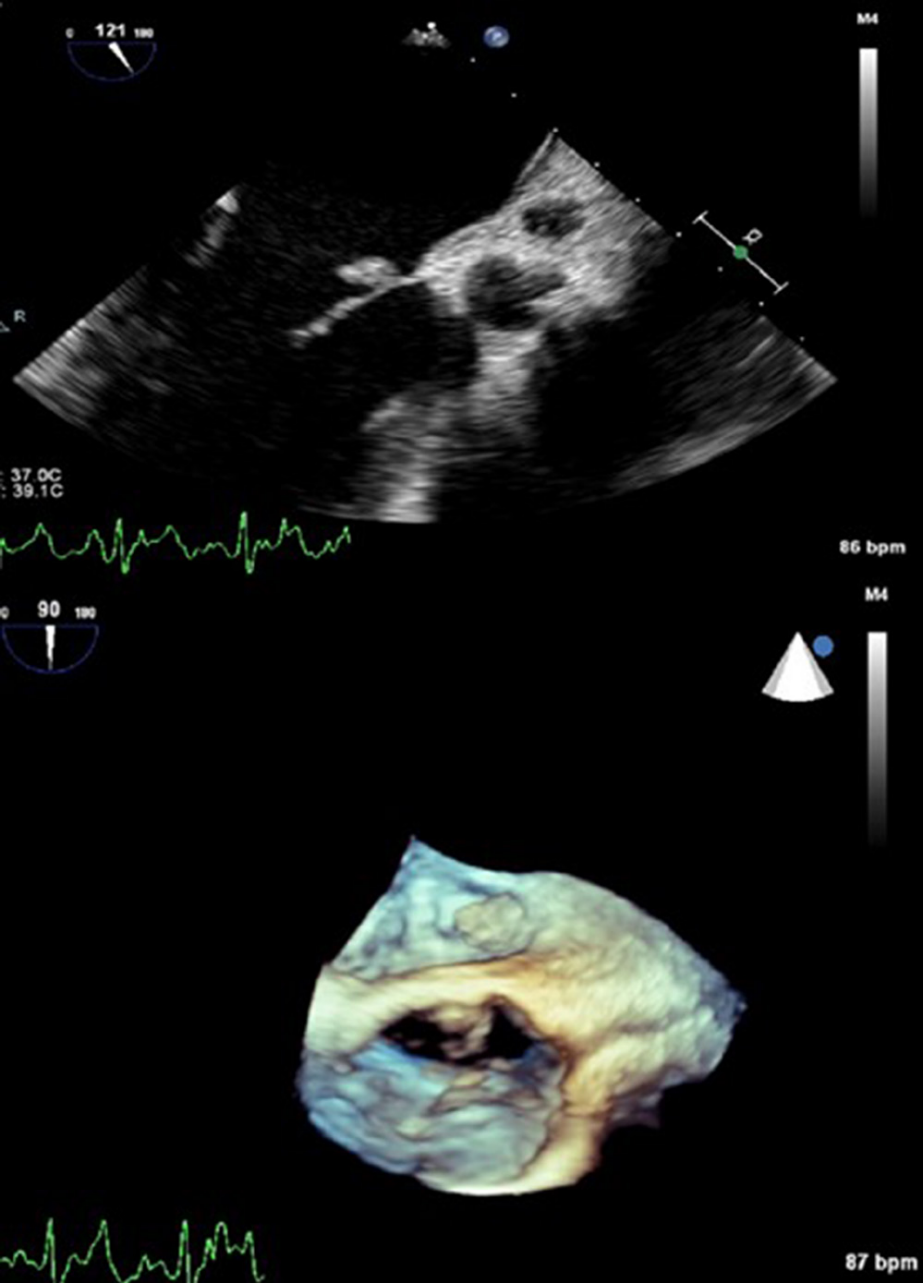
Transesophageal echocardiogram images of the mitral valve revealing a 1.1×0.7 cm mobile vegetation.
